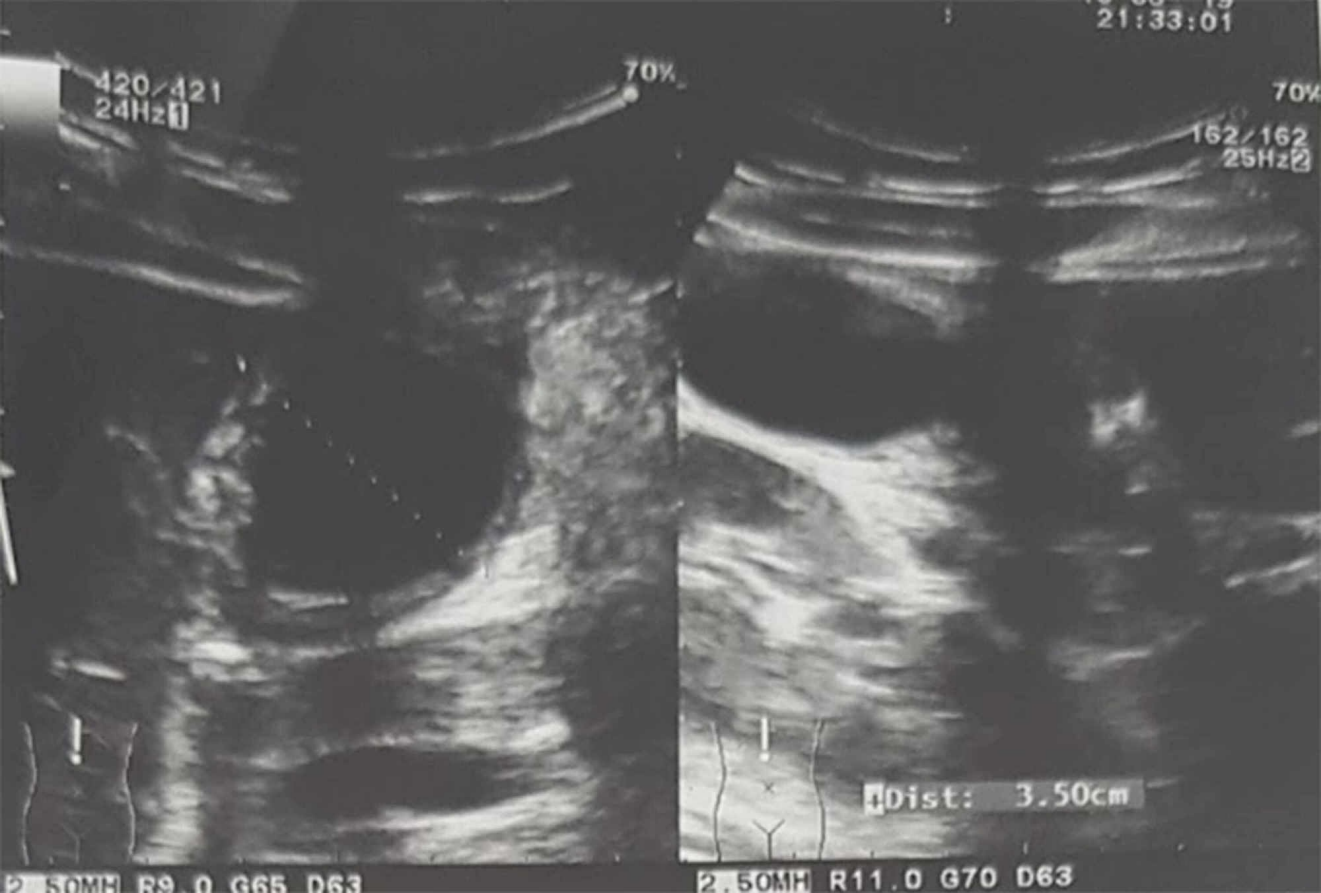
Sequential abdominal ultrasonography The ultrasonography showed a more marked distended gallbladder and thickened gallbladder wall
